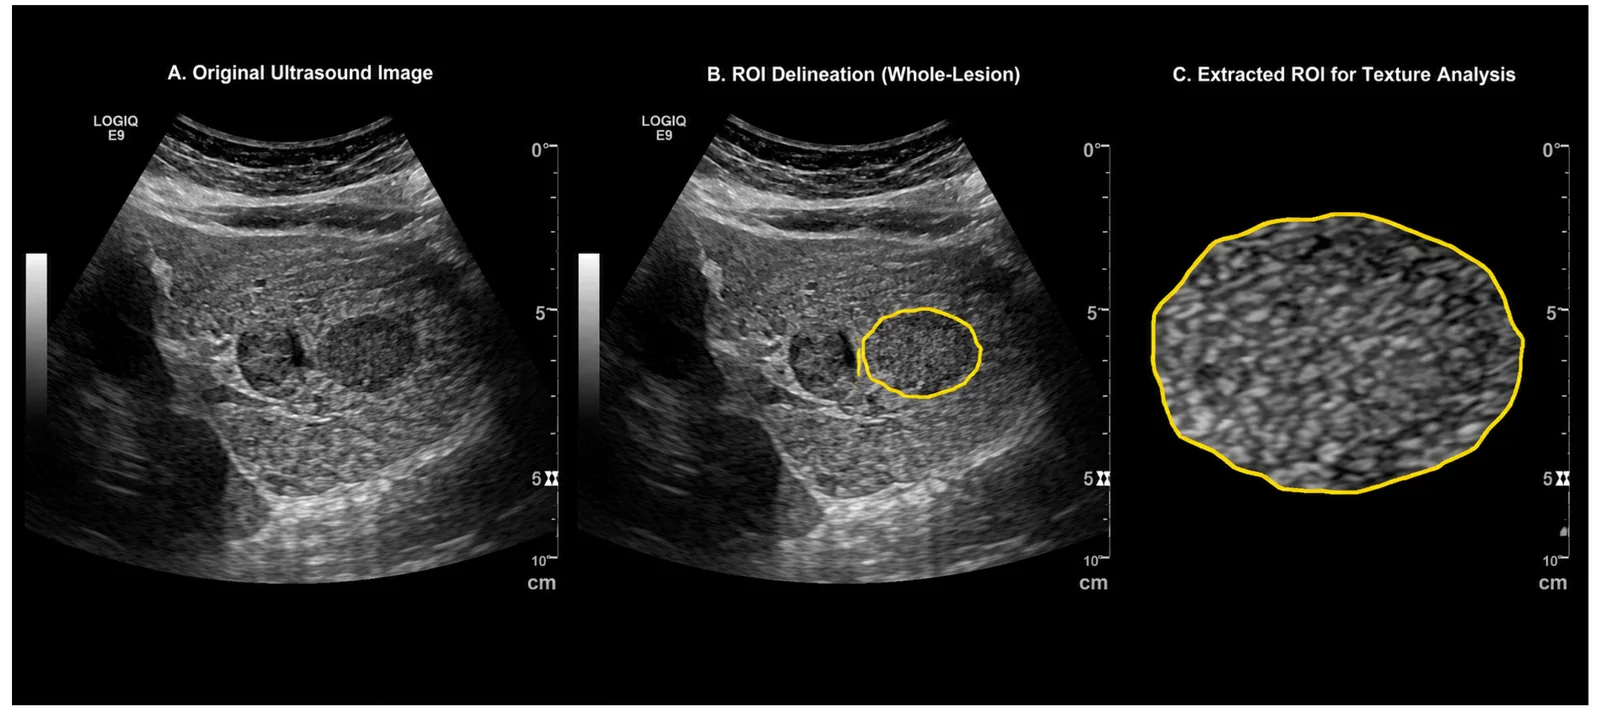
Ultrasound image acquisition and ROI-based texture analysis of a hepatic metastatic lesion. Representative grayscale ultrasound image of a liver metastasis (left), manual delineation of the lesion by whole-lesion ROI segmentation (middle), and isolated ROI used for texture feature extraction and quantitative tissue analysis (right).
